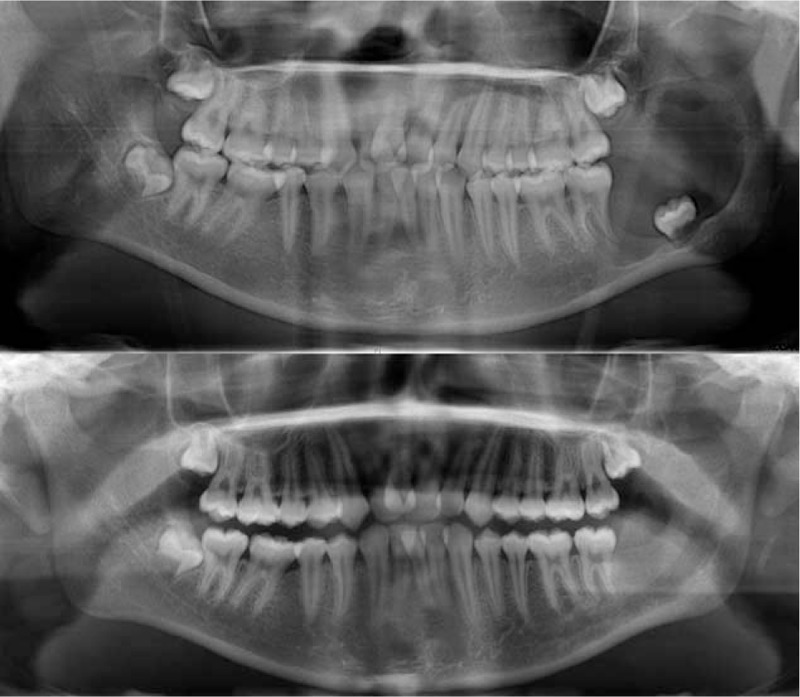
The radiographs (A) before marsupialization and (B) 8 months after marsupialization.
